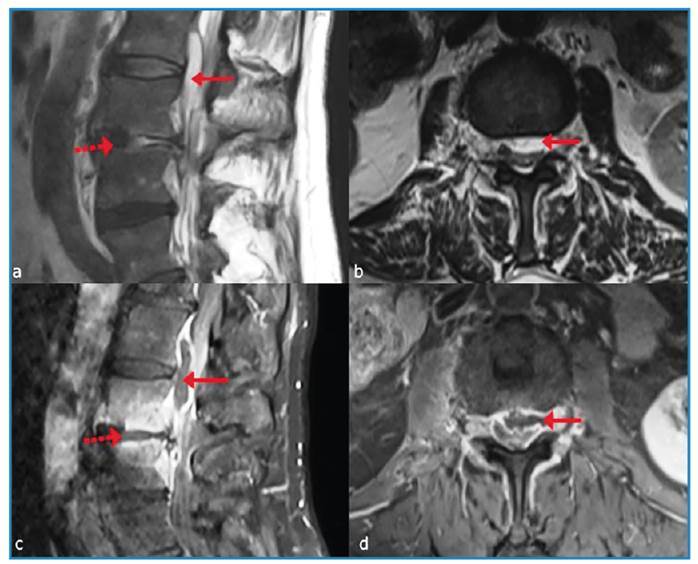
Pre-treatment magnetic resonance imaging showing tuberculous spondylodiscitis and epidural abscess. (a, b) Sagittal and axial T2-weighted images with fat suppression (T2 AG) show hyperintense signal intensity in the L3-L4 disc space (dotted arrow), indicating spondylodiscitis. Additionally, an abscess formation is visualized with a long arrow. (c, d) Sagittal and axial T1-weighted images with fat suppression and contrast enhancement (T1 AG) demonstrate contrast enhancement in the L3-L4 disc space and vertebral endplates (dotted arrow), suggesting active infection. The peripheral rim of the abscess formation also shows contrast enhancement (long arrow).
